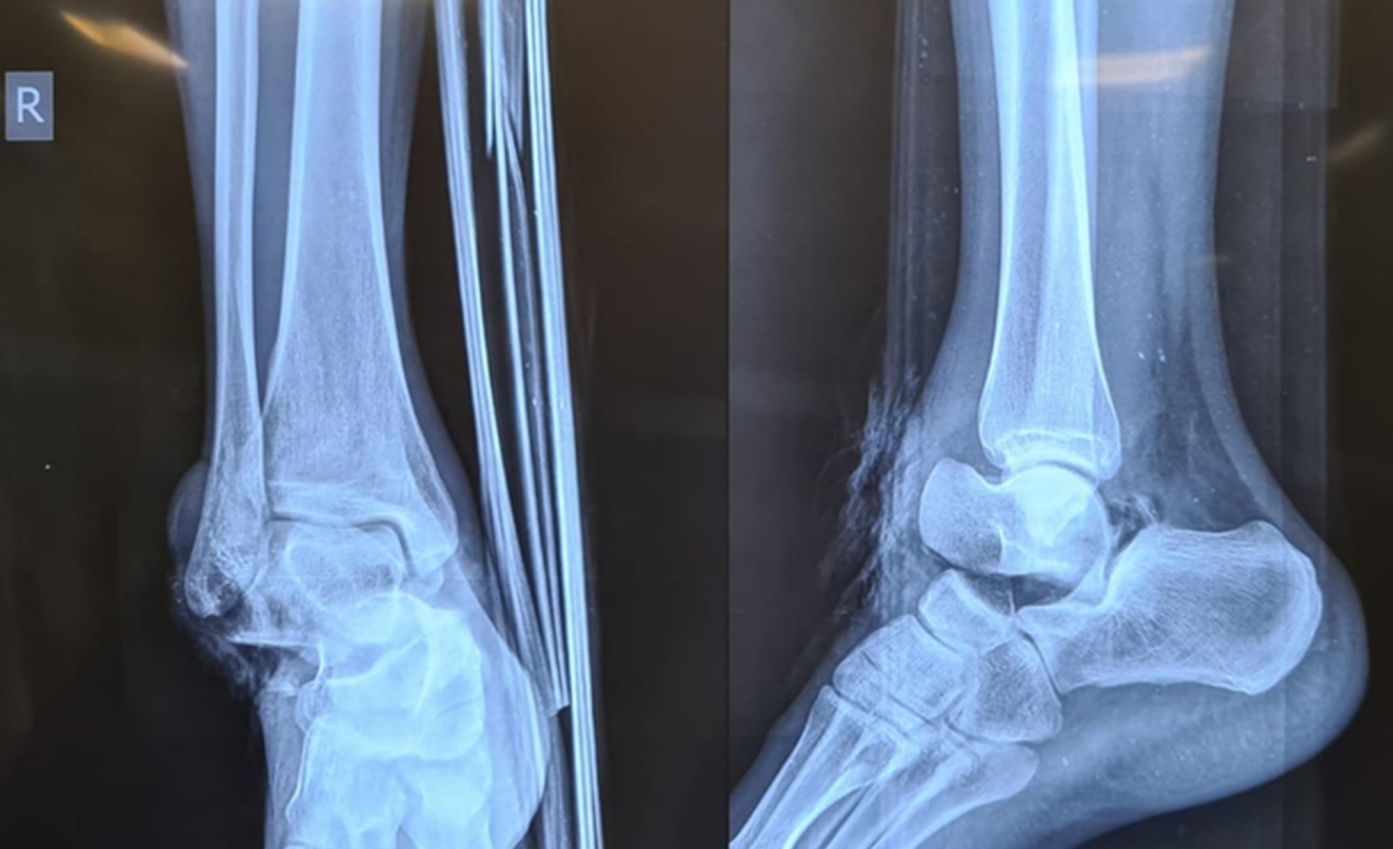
Plain radiograph of the right ankle (anteroposterior and lateral views) showing medial subtalar dislocation. The lateral view shows that the talar head is superior to the navicular.
